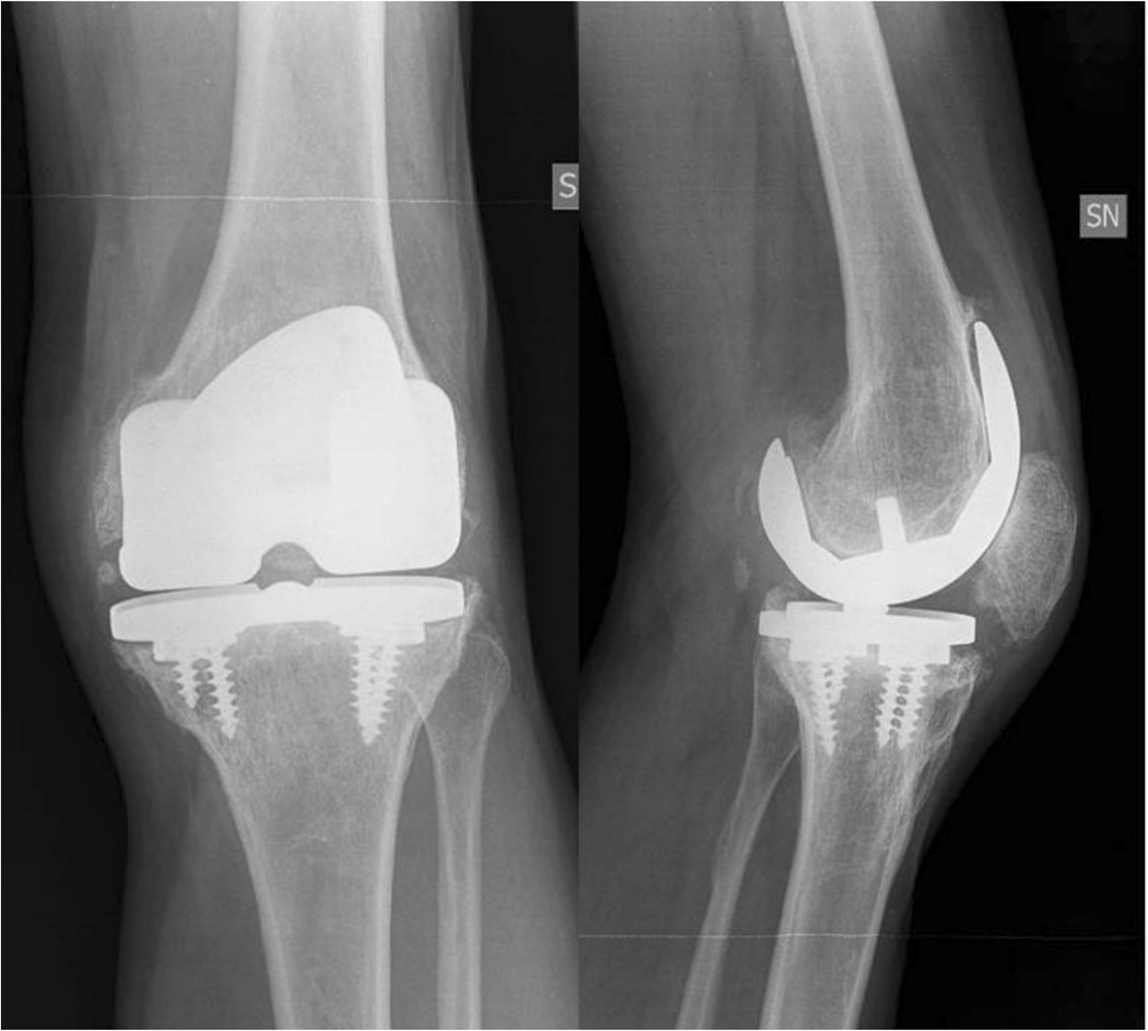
Preoperative radiographs showing the original knee implant following the arthrodesis with clear radiolucency.
